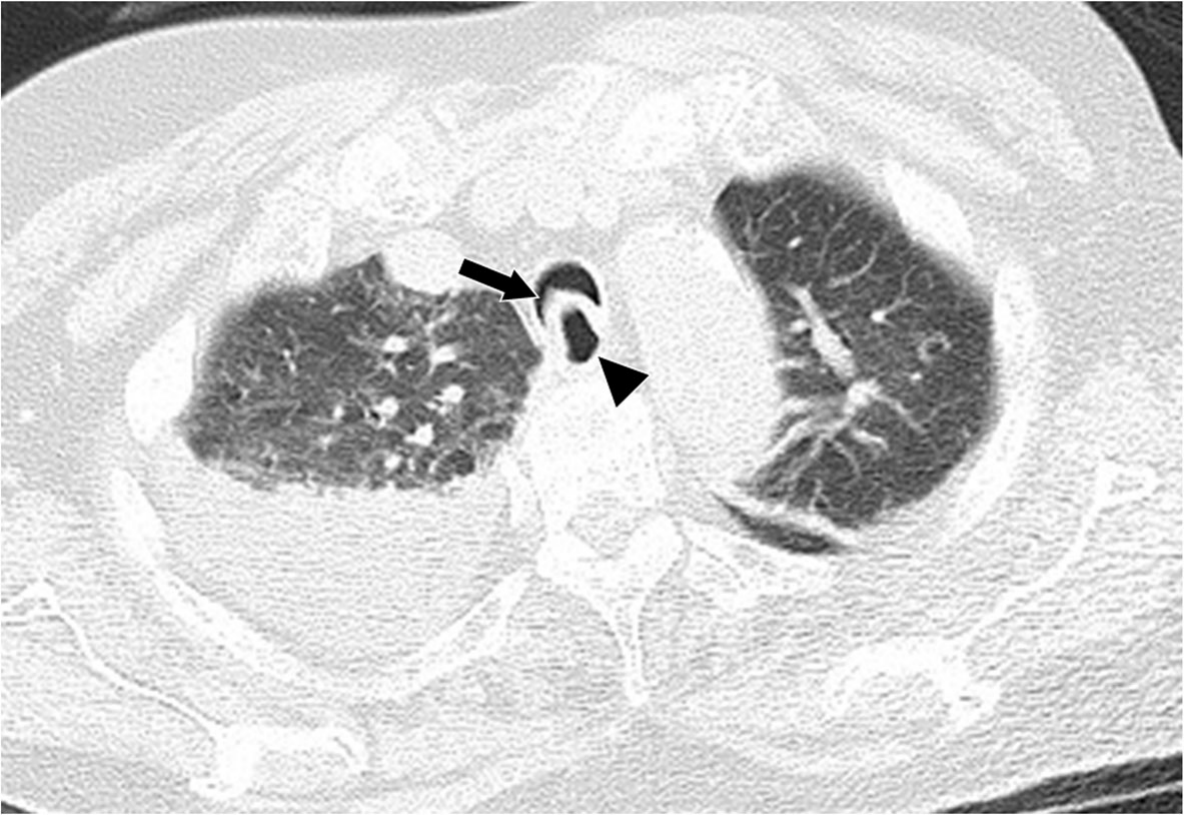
Computed tomography scan of the chest. Computed tomography scan of the chest taken before surgery showing bulging of the posterior membrane (allow) into the airway lumen without collapse of the cartilage at the aortic arch level. An arrowhead indicates the esophagus
